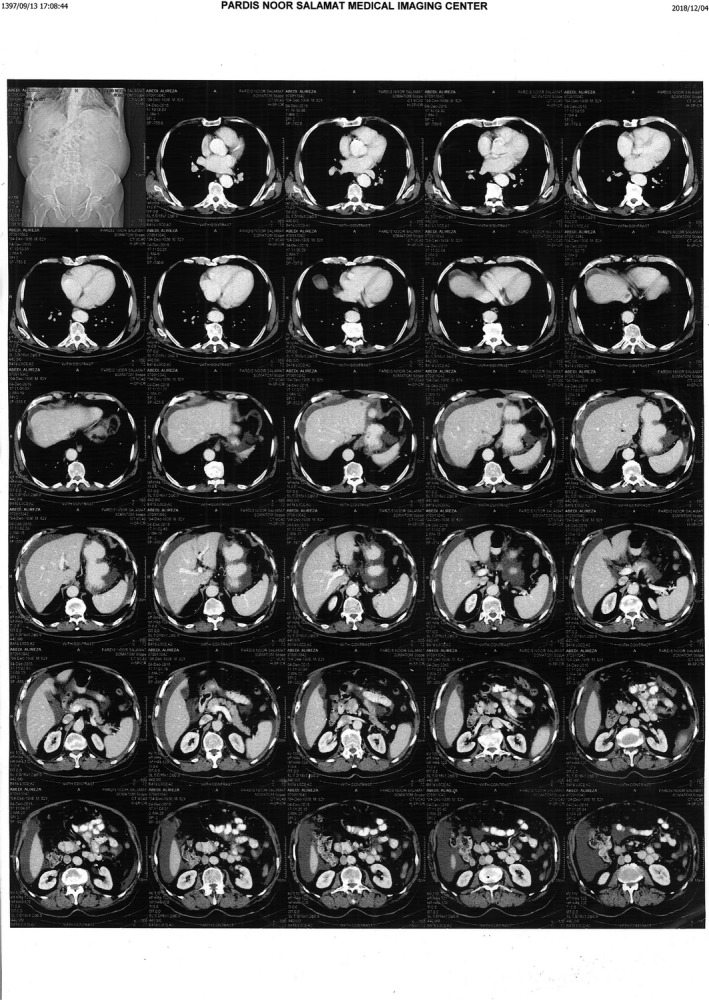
The first CT Scan of the patient on 30/11/2018
